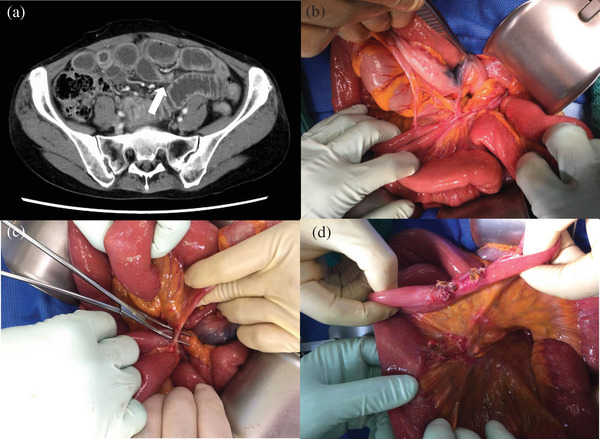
Abdominal computed tomography and operative findings. (a) The computed tomography examination revealed a caliber change in the small intestine and a dilation of the oral intestinal tract. (b, c) Serosal ink was observed in the sigmoid colon. Near the ink, the small intestine is obstructed by fibrosis and complex bands caused by partial inflammation. (d) The ileum shows stenosis caused by inflammation
